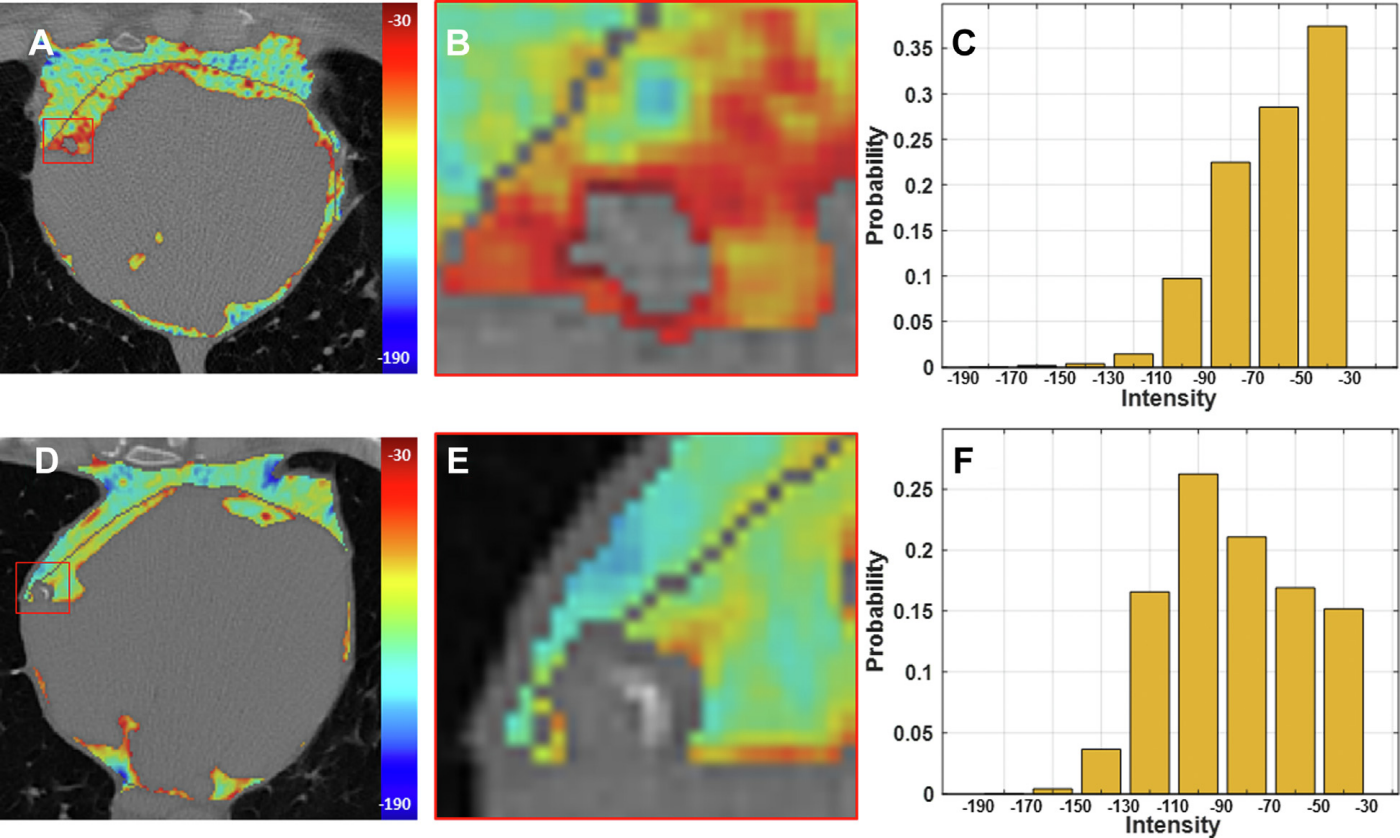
Example EAT CT Intensities (HU Values) in Patients With and Without MACE (A and D) EAT HU (inside the pericardium in red) is elevated as compared to PAT HU (outside the pericardium). Zoomed views show that the specialized EAT around the RCA (called PCAT) has elevated HU as compared to PCAT outside the black pericardium boundary. Importantly PCAT is clearly elevated in E relative to B. (C and F) Present normalized HU distributions for EAT. For the MACE patient (C), There is a conspicuous shift towards higher HU values with 37% of values within the [-50, -30 HU] range, as compared to 15% for the no-MACE patient.
